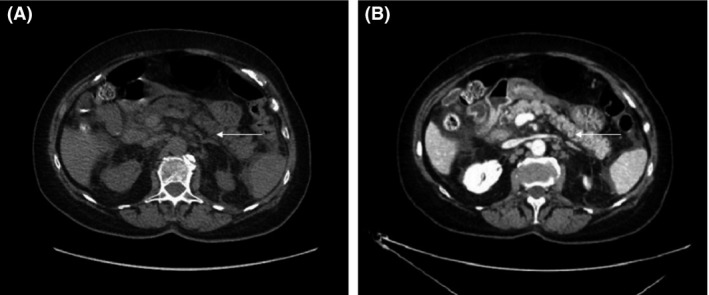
Computed tomography without (A) and after injection, early portal phase (B). Slight enlargement of pancreas (white arrows) and moderate infiltration of peripancreatic fat.
